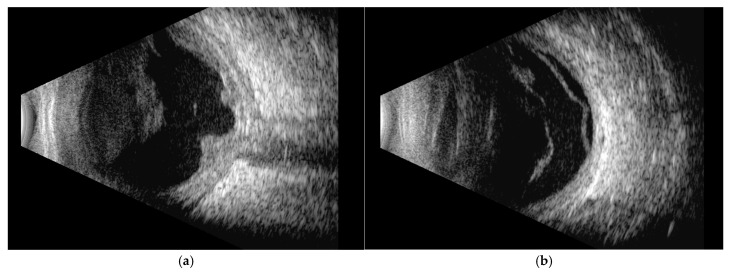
B-scan ultrasonography showing: (a) fibrotic consolidations and organized vitreous hemorrhage; choroidal thickening, alteration of the normal contour of the optic disc head, tram-track sign of the optic nerve sheath and hypoechogenicity of the retroscleral tissue. These findings are suggestive of optic nerve sheath and choroidal infiltration rather than hemorrhagic changes; (b) exudative retinal detachment.
